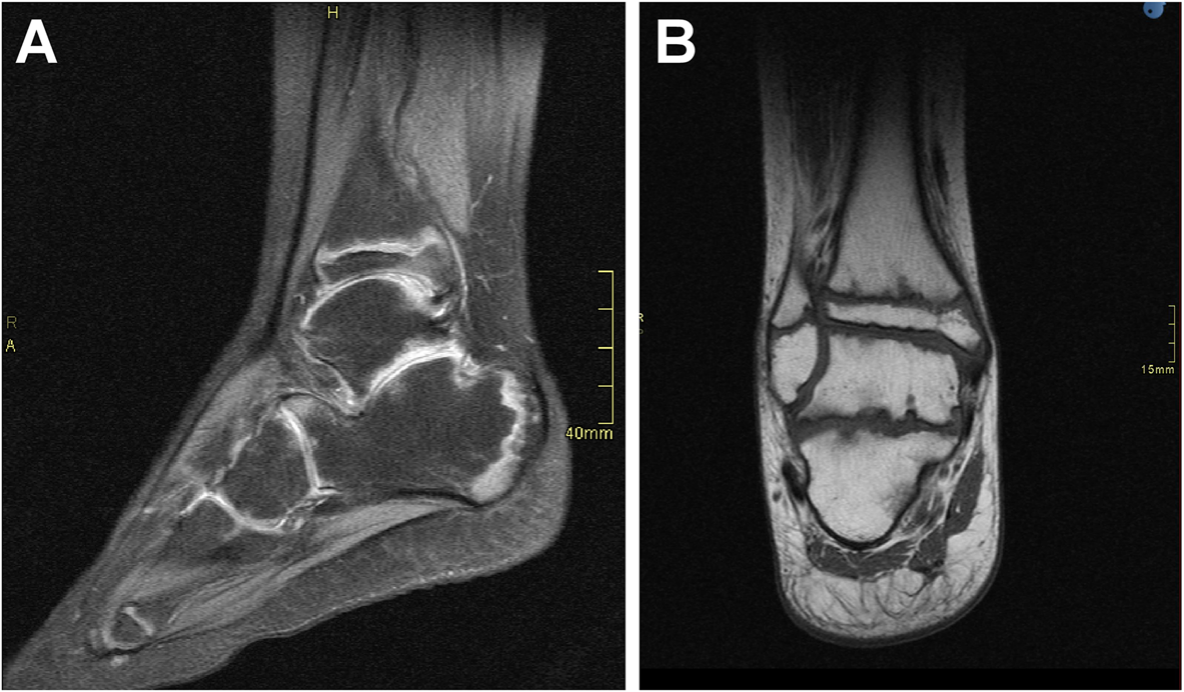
MRI scans performed elsewhere for the ankles (a and b) revealed the presence of arthritic changes in the form of irregularity in opposing joint surfaces, erosions, and narrow joint spaces with patchy areas of bone marrow edema
